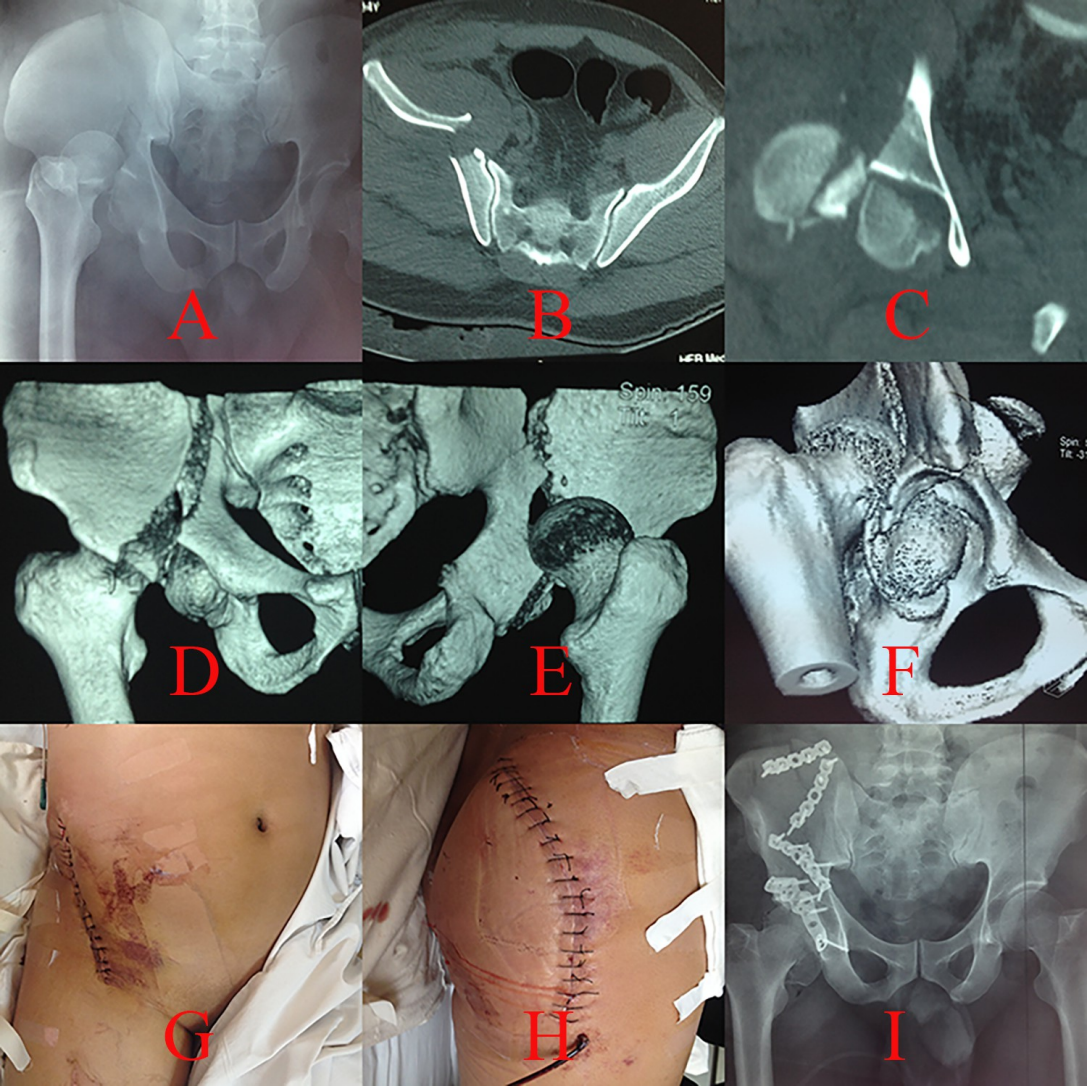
A patient with A3.3 injury managed through combined approaches was presented. A, cephalad detachment of roof column and femoral head with an intact iliopectineal line; B-C, detachment of roof column/wall and femoral head on CT scans; D-F, cephalad detachment of roof column/wall and femoral head dislocation on 3D reconstruction; G-H, surgical incisions (iliac fossa and KL); I, imperfect reduction quality on postoperative AP view.
